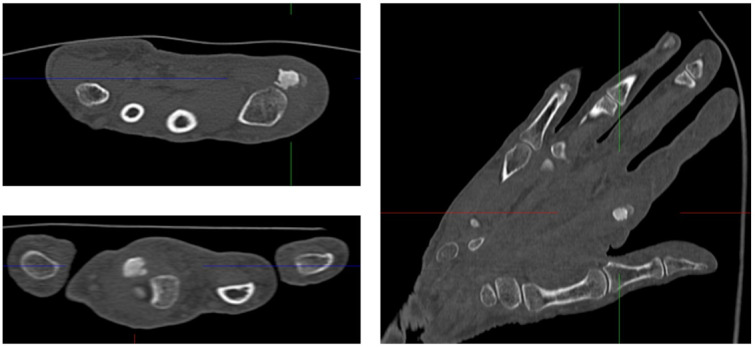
Preoperative Computed Tomography (CT) of the right hand
